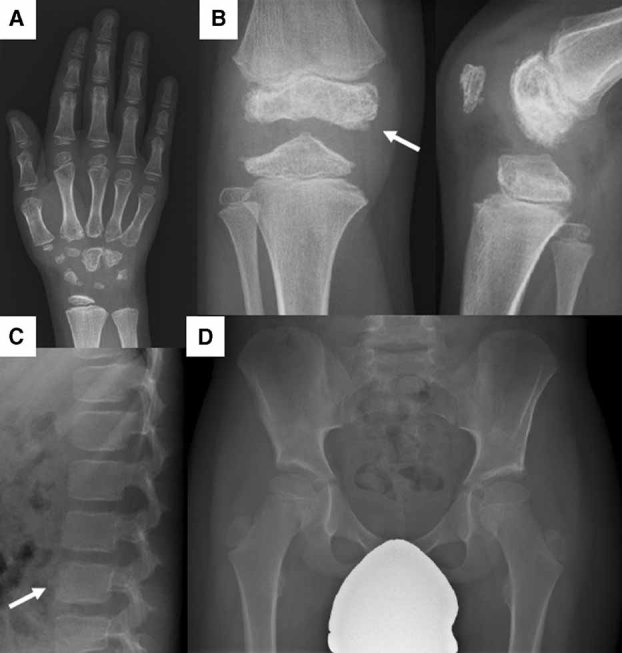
Radiographs of the proband in Family 2 (individual III-1) at age 9 years and 10 months of age showing (A) hand, (B) knee, (C) spine, and (D) hips. The radiographs were consistent with a diagnosis of MED, characterized by delayed and abnormal endochondral ossification. The hand showed marked carpal ossification delay with irregular carpal bones with a coarse internal structure. The distal epiphysis of the radius was also irregular and small. The knees had small and irregular femoral and tibial epiphyses and irregular metaphyses. The pelvis showed some flattening of the proximal femoral epiphyses but they were relatively spared. There was some irregularity of the vertebral end plates (arrow).
